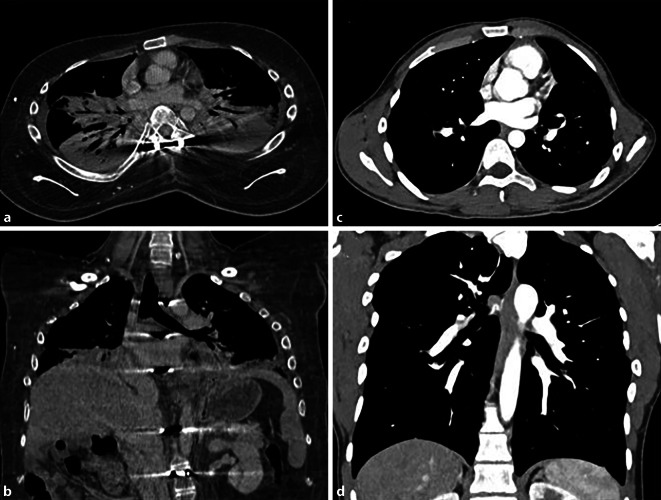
a, b CT-Thorax eines 23-jährigen Patienten mit Duchenne-Muskeldystrophie (a axiales Weichteilfenster, b koronale Rekonstruktion). c, d CT-Thorax eines gleichaltrigen, gesunden jungen Mannes (c axiales Weichteilfenster, d koronale Rekonstruktion). Im direkten Vergleich wird die schwere Atrophie der Skelettmuskulatur deutlich, einschliesslich der für die Atmung essenziellen Interkostalmuskulatur (a, b). Auffällig zudem die sekundäre Volumenreduktion der Lunge mit konsekutiv bilateralem Zwerchfellhochstand bei dem Patienten mit Duchenne-Muskeldystrophie (b). Im Vergleich dazu zeigt der gesunde Patient eine altersentsprechende Lungenkapazität mit deutlich tiefer stehenden Zwerchfellschenkeln (d). Zusätzlich finden sich in a bilaterale Konsolidationen in beiden Unterlappen, dies entspricht mutmaßlich begleitenden lobärpneumonischen Infiltraten. Diese Patienten neigen aufgrund der zunehmenden Schwäche der exspiratorischen Atemmuskulatur und der gleichzeitig reduzierten Effektivität des Hustens zu einem deutlich erhöhten Aspirationsrisiko. Rezidivierende Infektionen begünstigen im weiteren Krankheitsverlauf die Ausbildung postentzündlicher struktureller Parenchymschäden, was die Prädisposition für weitere Infektionen und somit das Risiko für Komplikationen weiter erhöht
